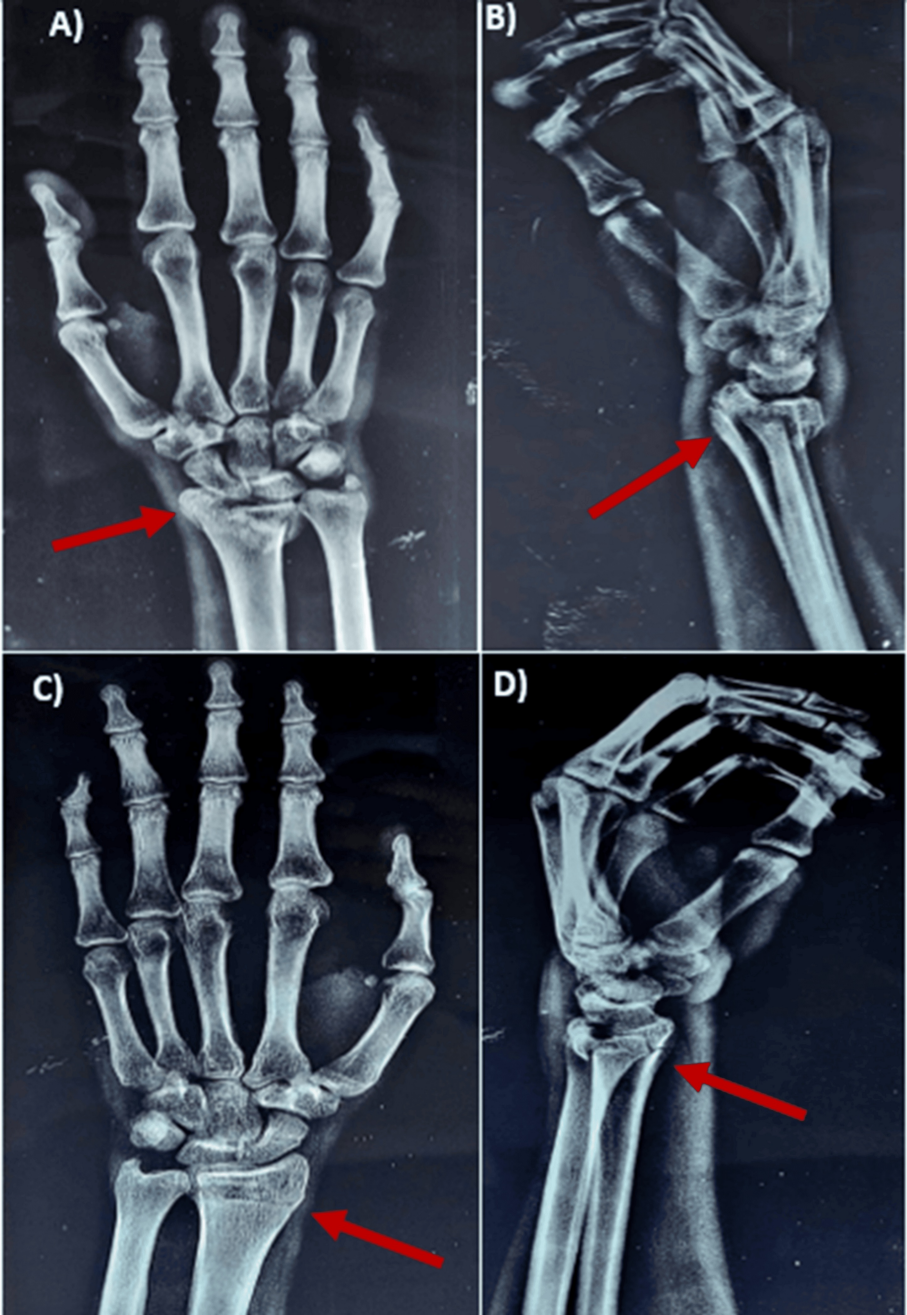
Preoperative X-rays A) Preoperative X-ray of the right wrist in AP view showing distal end radius fracture. B) Preoperative X-ray of the right wrist in lateral view showing distal end radius fracture. C) Preoperative X-ray of the left wrist in AP view showing distal end radius fracture. D) Preoperative X-ray of the left wrist in lateral view showing distal end radius fracture
